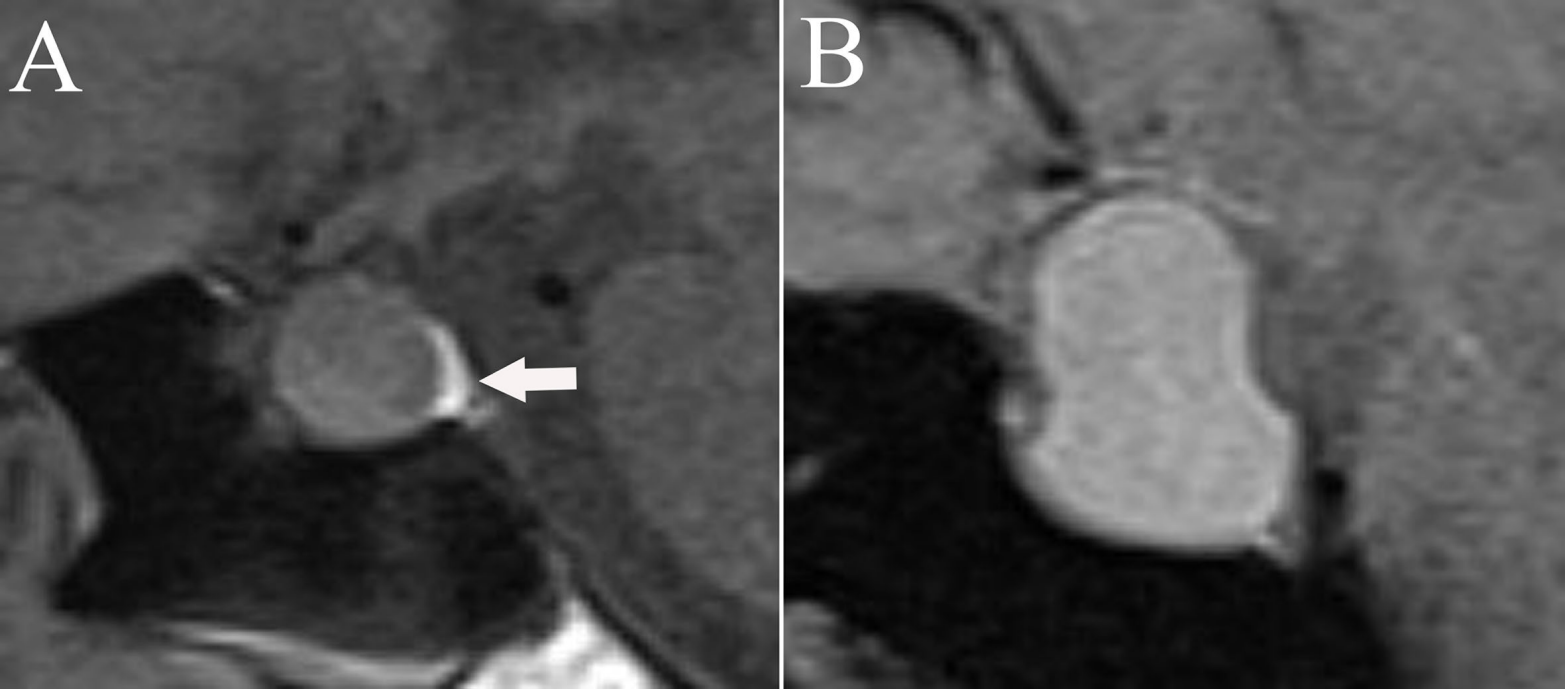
The appearance and location of posterior pituitary bright spots (white arrow) on T1-weighted images. (A) Posterior pituitary bright spots locates under the diaphragma sellae. (B) Posterior pituitary bright spots is nonvisable.
